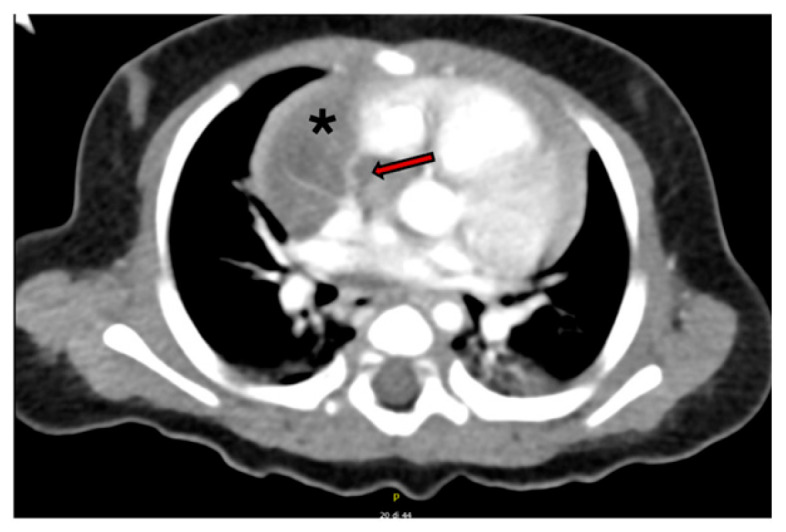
Angio- CT scan. Right paracardiac lesion with well-defined and regular margins, tightly adherent to the mediastinal structures. It indents the lateral wall of the right atrium and compresses the superior vena cava. Its average density is supraliquid in nature; however, within the lesion the presence of some vascular structures originating from the right pulmonary artery is confirmed. Red arrow: vascular pedicle, Asterisk: lesion.
